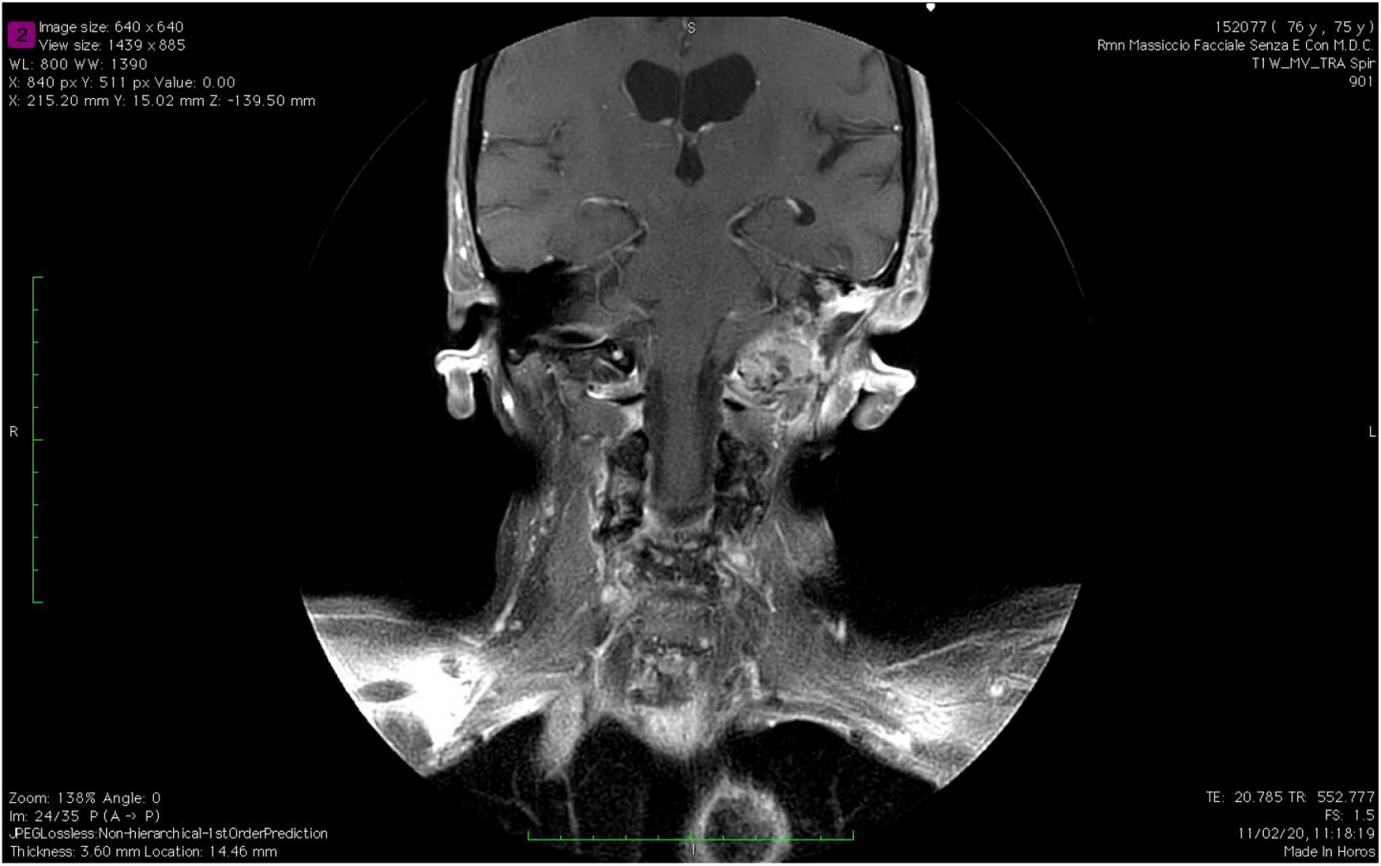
Sixteen months MRI follow up (coronal view). In the site of previous left petrosectomy the MRI scan showed an heterogeneously enhanced tissue; toward the rear, it grows through the foramen lacerum involving the foramen magnum.
